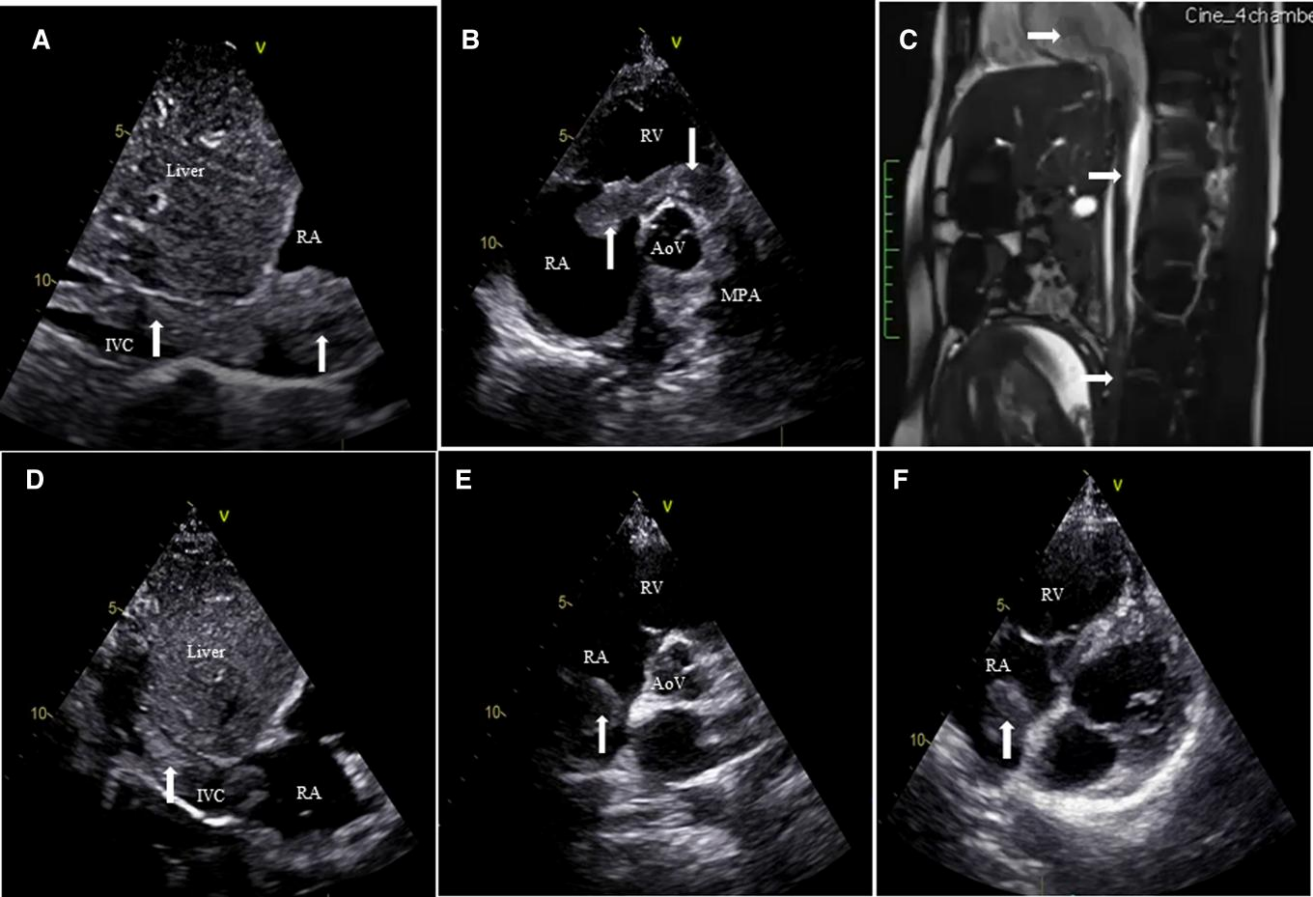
White arrows represent the clot. (*A*) Subcostal echo view showing serpiginous thrombus entering right atrium via inferior vena cava. (*B*) Parasternal short axis echo view—thrombus prolapsing across tricuspid valve into right ventricle and then across the pulmonary valve into main pulmonary artery. (*C*) Magnetic resonance imaging showing continuous filling defect from inferior vena cava to right atrium/right ventricle. (*D*) Subcostal inferior vena cava view showing decrease in the thrombus burden in inferior vena cava and right atrium after thrombolysis. (*E*) Parasternal short axis echo view showing resolution of clot in right ventricle and main pulmonary artery post thrombolysis. (*F*) Apical four-chamber view showing residual clot in right atrium.
